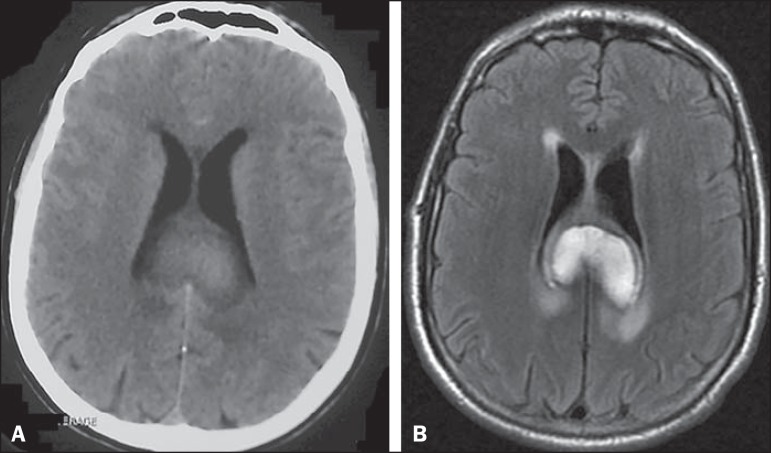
Non-contrast computed tomography (A) and T2-weighted fluid
